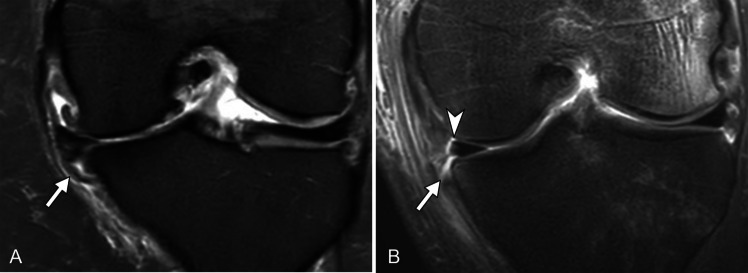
Meniscotibial ligament. Coronal proton-density-weighted fat-suppressed MRI of a 59-year-old woman (A) with complete tear of the posterior root of the medial meniscus and meniscal extrusion depicts medial meniscotibial ligament (arrow). Coronal proton-density-weighted fat-suppressed MRI of a 32-year-old man (B) with ACL tear demonstrates a tear of the medial meniscotibial ligament (arrow); note associated meniscocapsular tear (arrowhead)
